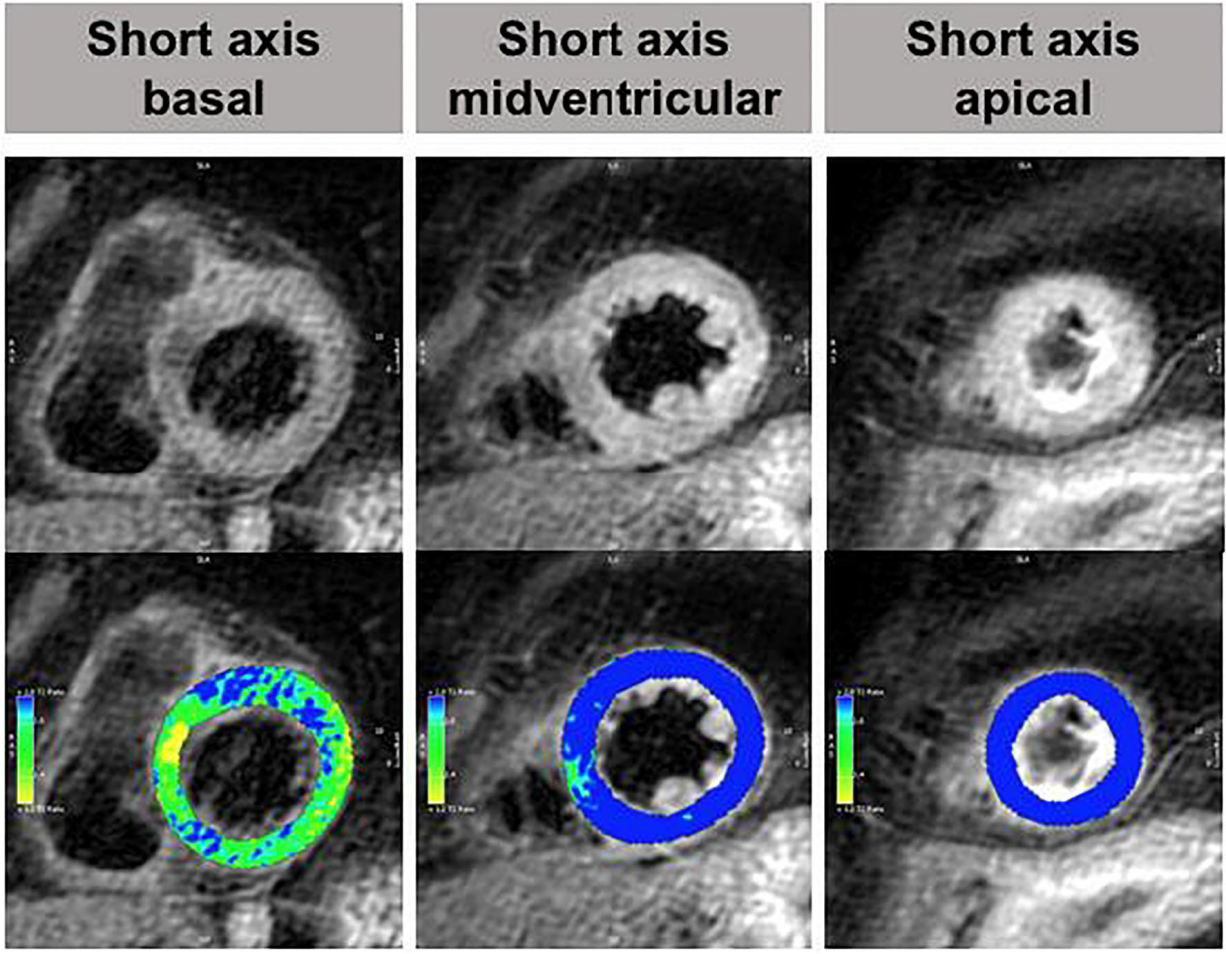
CMR imaging in a patient with typical apical TTS. Top row: T2-weighted short tau inversion recovery images demonstrating normal signal intensity of the basal myocardium but global oedema of the mid- and apical segments with impaired systolic function. Bottom row: Computer-aided signal intensity analysis of the oedema images (blue indicates a signal intensity ratio of myocardium to skeletal muscle ≥ 1.9 equivalent to oedema; green/yellow indicates a normal signal intensity ratio < 1.9)
